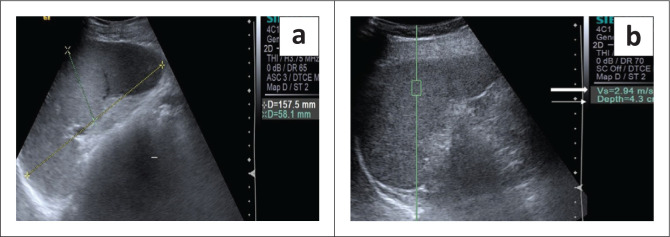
Splenic acoustic radiation force imaging (ARFI) evaluation in a control subject: Grey scale ultrasound (a) shows splenic longitudinal dimension taken as the maximum distance from upper to the lower pole at the level of splenic hilum (15.7 cm). (b) Shows ROI placement for splenic ARFI evaluation. The rectangular ROI was placed at a depth of 4.3 cm (arrow) from the skin surface in the splenic parenchyma devoid of blood vessels. Shear wave velocity (SWV) in this case was 2.94 m/s (solid arrow). Ten random SWV (in m/s) measurements were taken from the upper pole to the lower pole of the spleen during suspended respiration.
